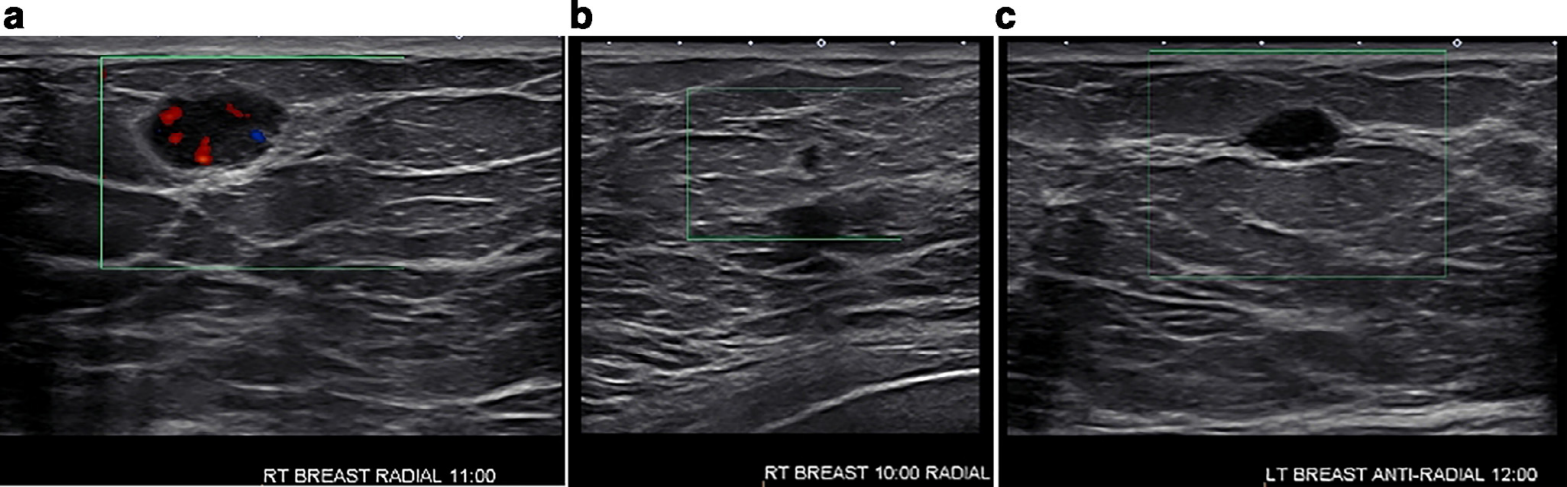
Diagnostic high resolution sonography of both breasts confirmed ultrasound correlates for the mammographic masses, all classified as BI-RADS 4. This included (a) targeted sonography with color Doppler demonstrating an oval, circumscribed, parallel oriented, hypoechoic 1.5 cm right breast mass with internal vascularity at 11-o'clock, 9 cm from the nipple, corresponding to the patient's palpable complaint, (b) an irregular, spiculated 0.4 cm right breast mass with anti–parallel orientation at 10-o'clock, 10 cm from the nipple, and (c) an oval, circumscribed, parallel oriented, hypoechoic 1.0 cm left breast mass at 12-o'clock, 6 cm from the nipple.
